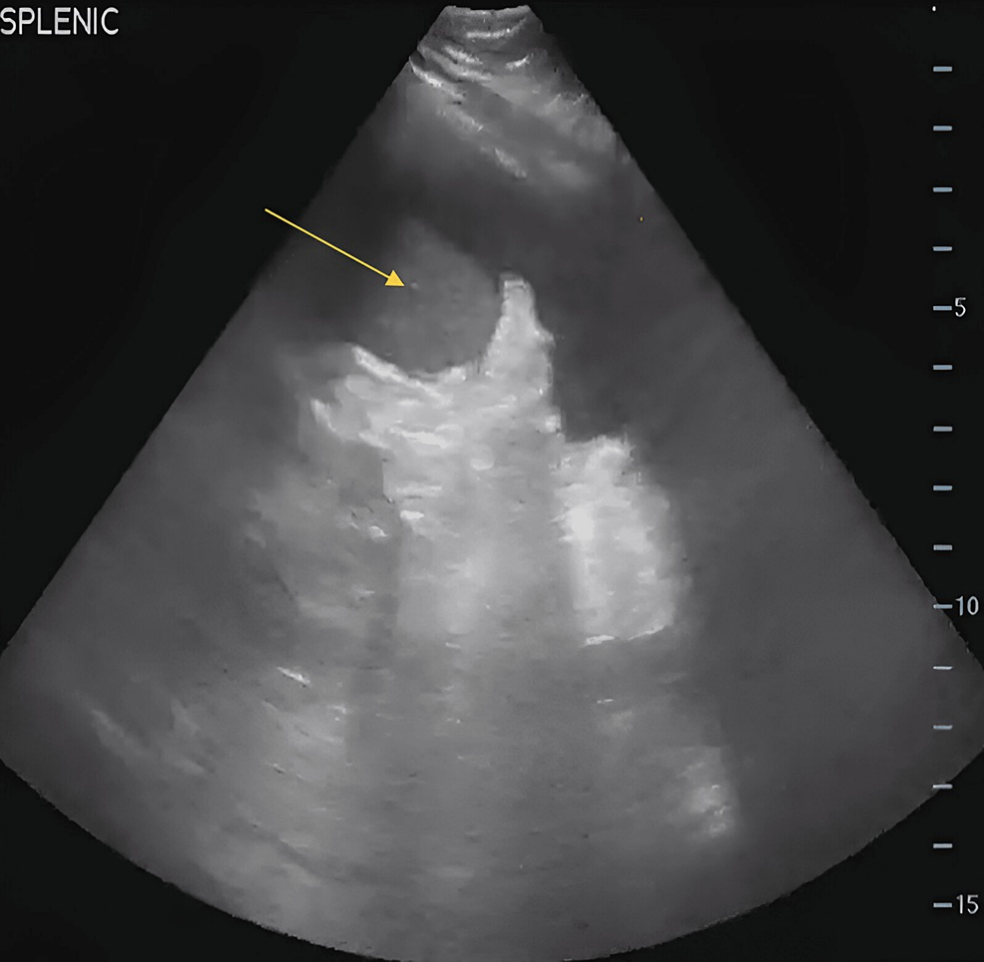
Abdominal ultrasound showing a hypoechoic lesion indicating subcapsular splenic hematoma/bleeding (yellow arrow)
